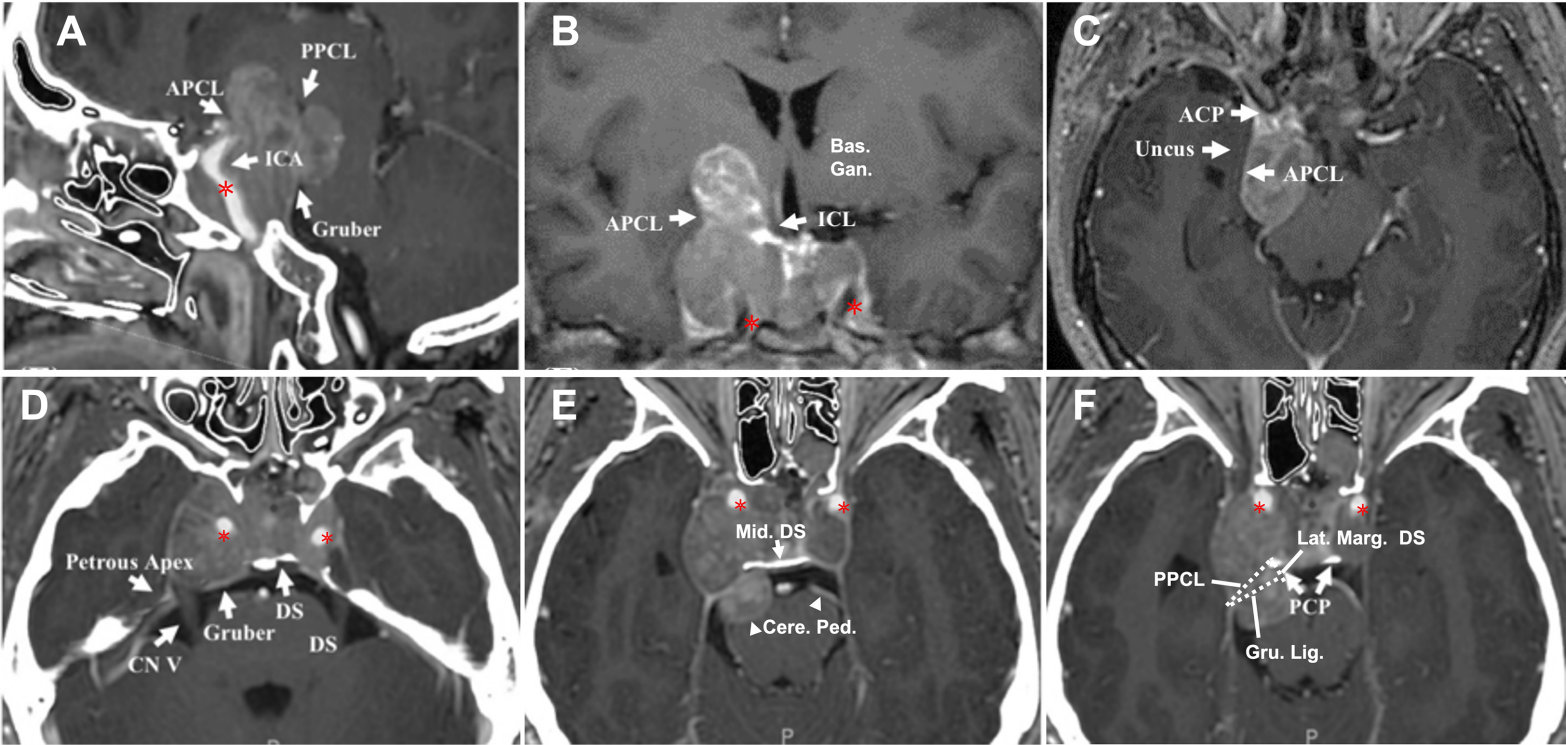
CASE3, The superior-posterior compartment extension. A 66-year-old woman with a giant pituitary adenoma. (A–C), Preoperative sagittal (A), coronal (B), and axial (C) T1-weighted contrast-enhanced MRI showing a pituitary macroadenoma with bilateral cavernous sinus invasion (Knosp grade 4) and extension through the superior, formed a “cauliflower-like” shape (A) and posterior (B) but not lateral (C) compartment. Note tumor expanded excess of the APCL (A, B) and ICL (B) superiorly and excess of the PPCL posteriorly (A) but was limited by APCL laterally (C). The superior aspect of the tumor compressed basal ganglia (B); the posterior-inferior aspect caused compression of the parapeduncular space (C). (D–F), Preoperative axial T1-weighted contrast-enhanced MRI at the dorsum sella root section (D); 5 mm above D at the middle dorsum sellar section (E); and 6 mm above (D) at the PCP section (F). (D) Intact dorsum sella, the petrous apex was seen, Gruber’s ligament is attached to the lateral dorsum sella, without displacement of bilateral CN V. (E) Posterior aspect of tumor excess posterior compartment dorsally of the Gruber’s ligament and compressed the ventral aspect of the cerebral peduncle (arrowhead) directly. (F) The typical pattern of posterior triangle invasion, which is excessing the triangle composed of PPCL superolateral, Gruber’s ligament superior, and the lateral margin of the dorsum sellae medially. Note the intimate relationship between ICA (asterisk) and the anteromedial aspect of the tumor. ACP, anterior clinoid process; APCL, anterior petroclinoidal ligament; DS, dorsum sellae; ICA, internal carotid artery; ICL, interclinoidal dural ligament; PCP, posterior clinoid process; PPCL, posterior petroclinoidal ligament; bas. gang., basal ganglia; cere. ped., cerebral peduncle; Gru. Lig., Gruber’s ligament, petrosphenoidal ligament; lat. marg., lateral margin; mid., middle.
